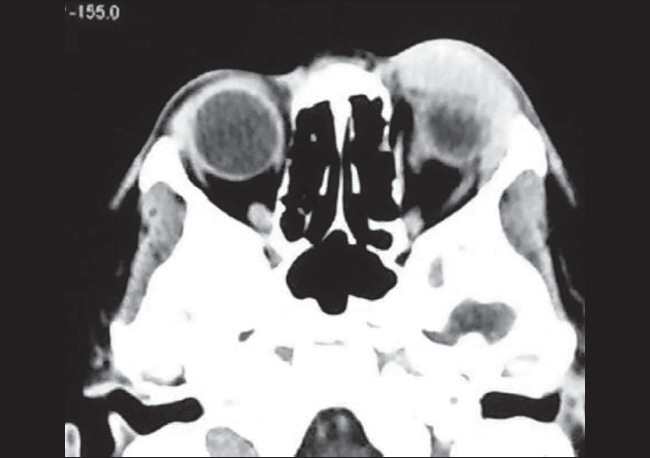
Computed tomography scan in axial view showing a soft tissue enhanced irregular mass in the antero-lateral aspect of the left orbit without evidence of bone erosion and defect
